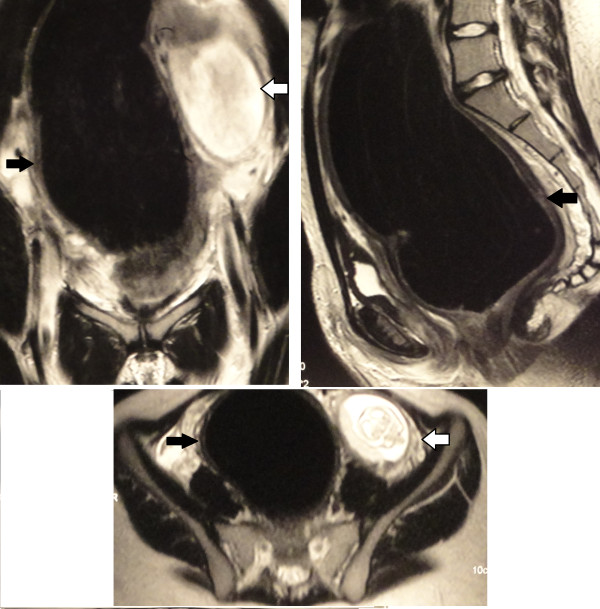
Magnetic resonance images showing megarectum and megasigmoid (black arrows) displacing the pregnant uterus to the left (open arrow).
